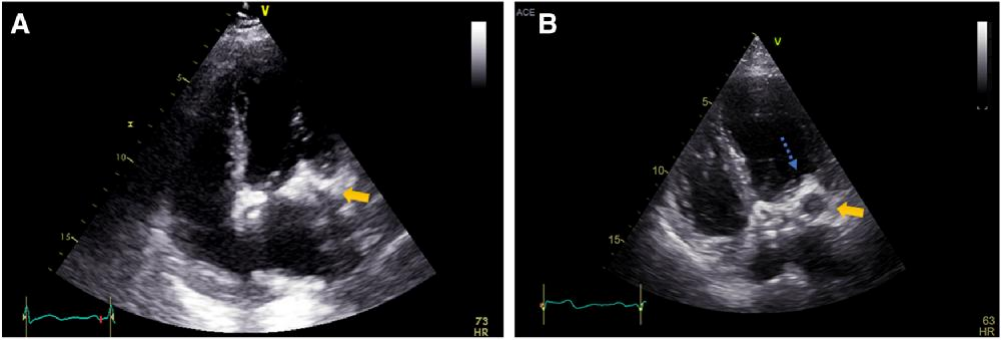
Transthoracic ultrasound, 4-chamber view, during the first and second hospitalizations (A and B, respectively). A hyperechoic cardiac mass on the mitral annulus is demonstrated in both studies (yellow solid arrows), with a clearing in the interior part of the mass, and an appearance of small mobile vegetation (blue dotted arrow), in the latter.
